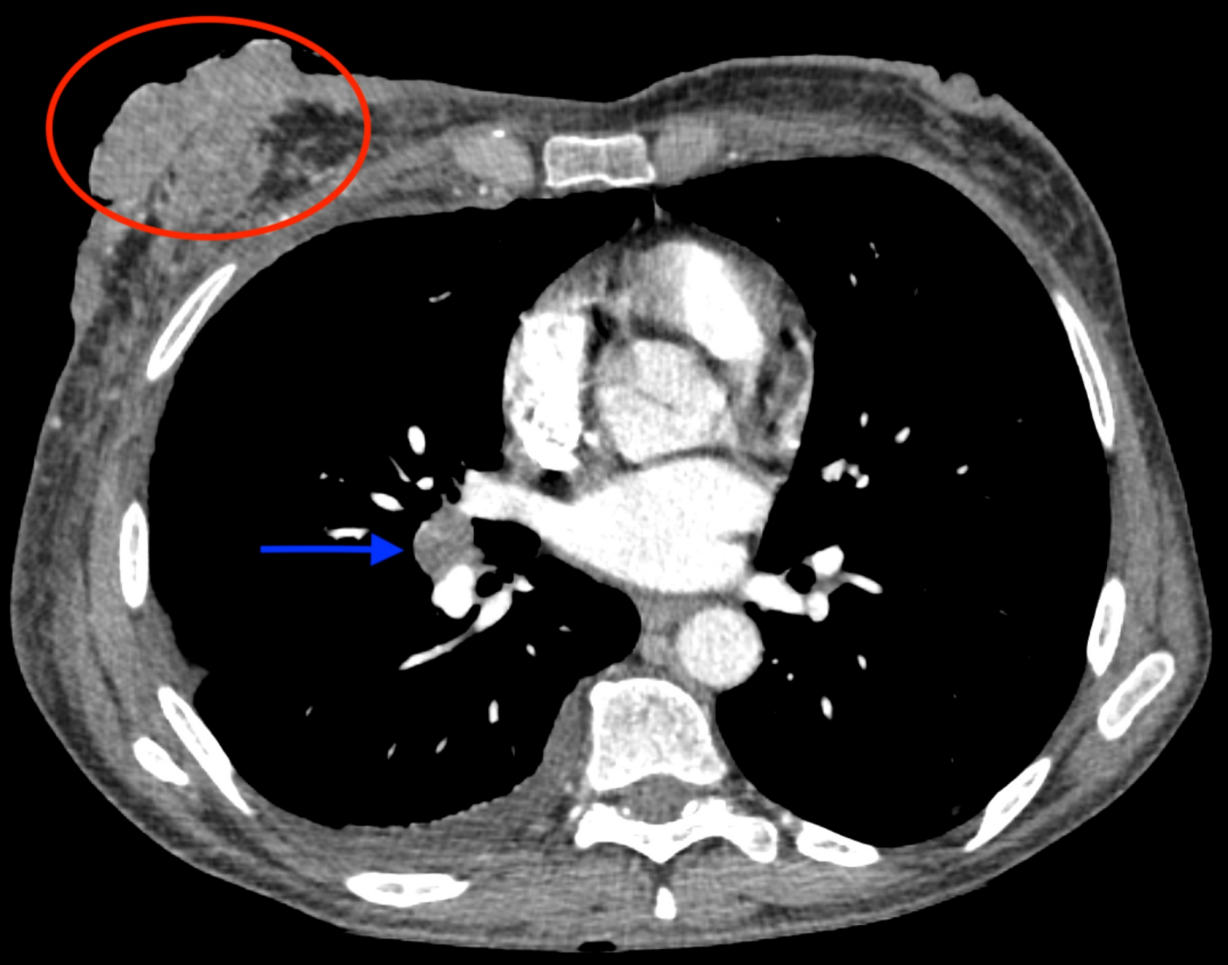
Axial image of the CT scan of chest at the level of nipples shows a large right breast mass (red circle) and right hilar lymphadenopathy (blue arrow)
